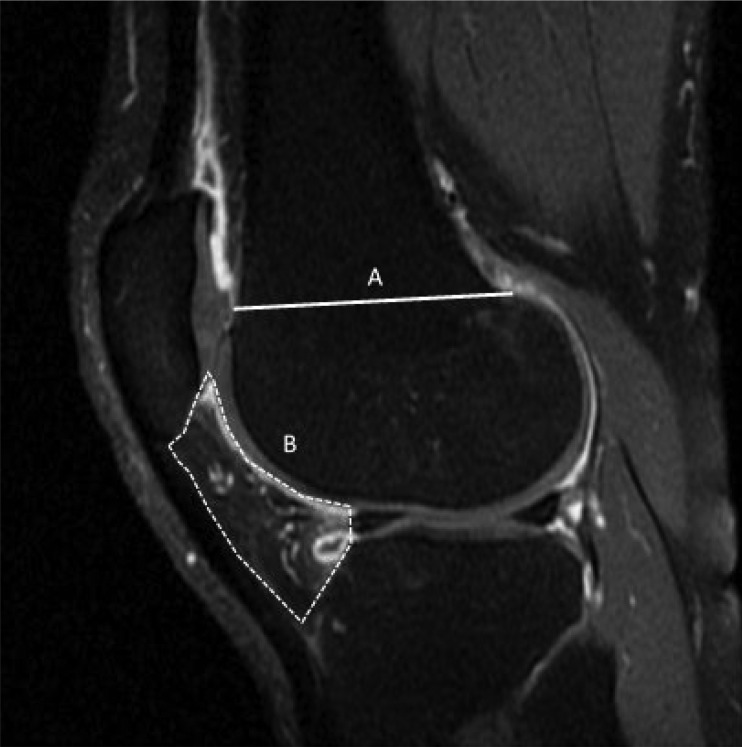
Sagittal T_1_-w fat suppressed (FS) contrast-enhanced MRI of a symptomatic osteoarthritic knee (A) Delineation of the femur for femoral condyle width measurement and (B) region of interest defined for the infrapatellar (Hoffa’s) fat pad.
